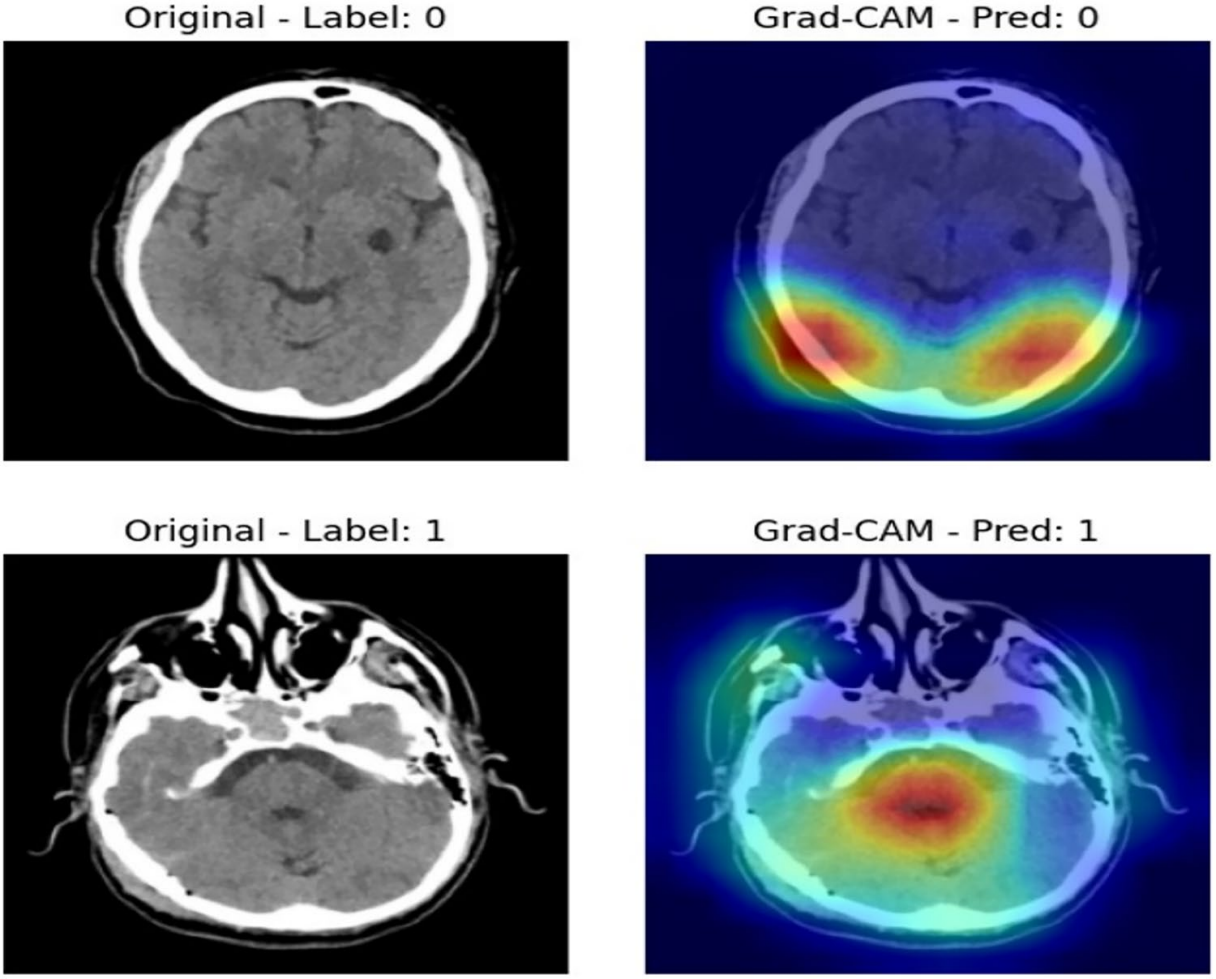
Highlighted regions indicate areas of the CT scan that influenced the model’s decision.
